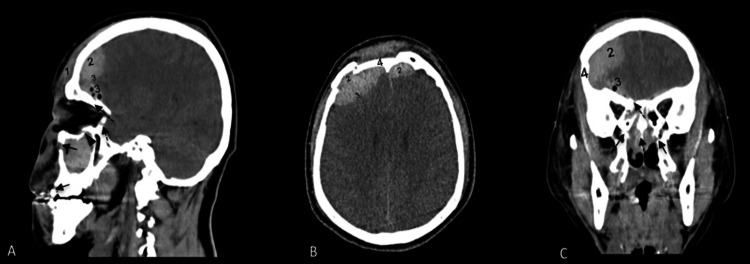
Initial non-contrast cranial and maxillofacial CT demonstrating severe traumatic brain injury associated with complex craniofacial fractures (A) Sagittal CT showing right frontoparietal subgaleal hematoma, with associated epidural and subdural hematomas in the setting of complex craniofacial fractures. (B) Axial CT demonstrating acute epidural and subdural hematomas along the right convexity, with a depressed right frontal bone fracture. (C) Coronal CT confirming a depressed frontal fracture with anterior cranial vault involvement. Maxillofacial CT shows a Le Fort II pattern, right orbital floor and medial wall fractures, and a type III NOE fracture. Annotations: (1) subgaleal hematoma; (2) epidural hematoma; (3) subdural hematoma; (4) depressed frontal fracture; arrows: Le Fort II components; arrowheads: orbital floor fracture; dashed arrows: NOE fracture. CT: computed tomography; NOE: naso-orbito-ethmoidal
